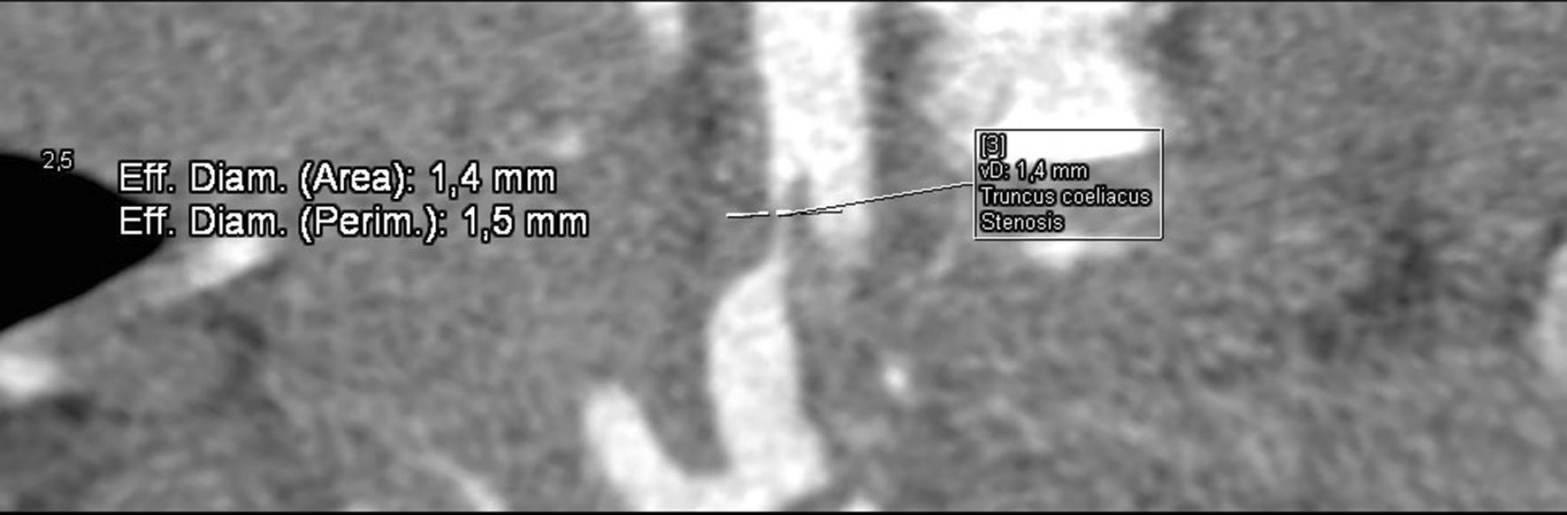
Computed tomography angiography (CTA), maximum intensity projection (MIP) reconstruction. Stenosis of celiac trunk to the diameter of 1.4 mm
